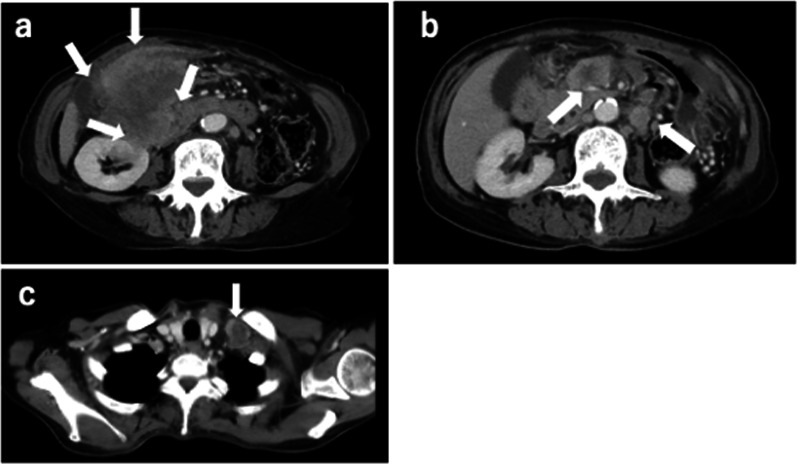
Computed tomography scan. a A tumor in the right transverse colon, directly invading the right kidney. b, c Swelling of the periaortic (b) and left supraclavicular lymph nodes (c) (arrows)
